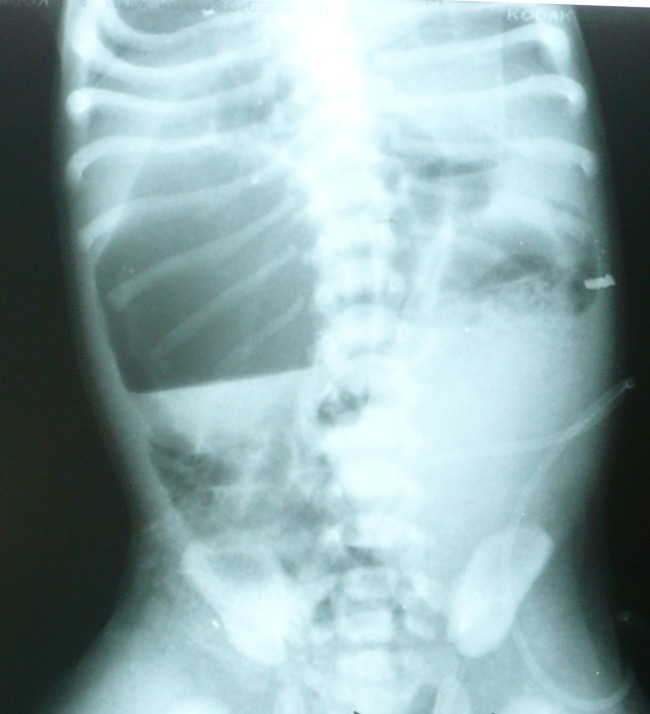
Figure 1: Abdominal radiograph is showing a big gas shadow in the right abdomen and ground glass appearance in the left abdomen. (The gas shadow represents proximal CSDC and ground glass portion may represent distal CSDC- as gas could not have reached the distal CSDC)
